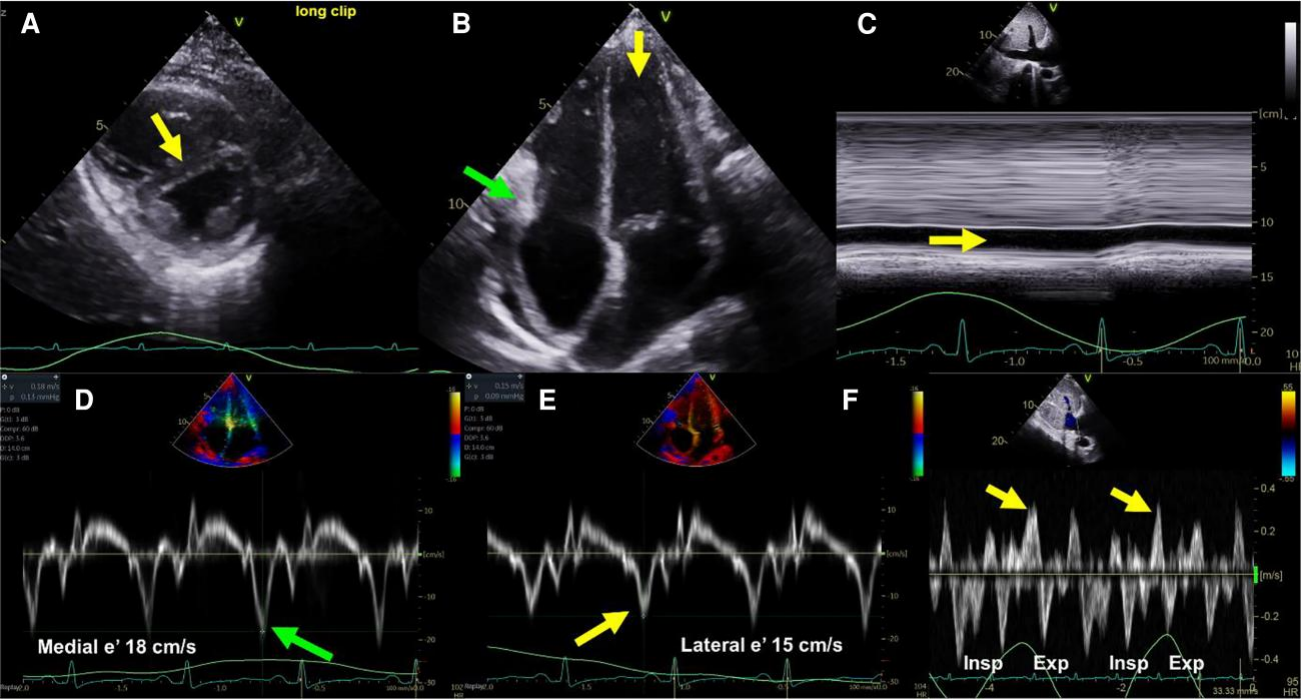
Echocardiography features of constrictive pericarditis. (*A*) Respirophasic interventricular septal motion abnormality, with leftward deviation and flattening of the septum during inspiration (yellow arrow). (*B*) Cylindrical deformity of the left ventricle (yellow arrow), and right ventricular lateral wall calcification and tethering (green arrow). (*C*) Inferior vena cava dilated (>2.1 cm) with minimal collapse <50 % with respiration. (*D*) and (*E*) shows mitral annular tissue Doppler e' velocity of 15 cm/s lateral (yellow arrow) and 18 cm/s medial (green arrow) indicating annulus reversus. (*F*) Increased end-diastolic reversal velocity of hepatic vein (yellow arrows) during expiration (>0.80 of forward velocity).^3^
